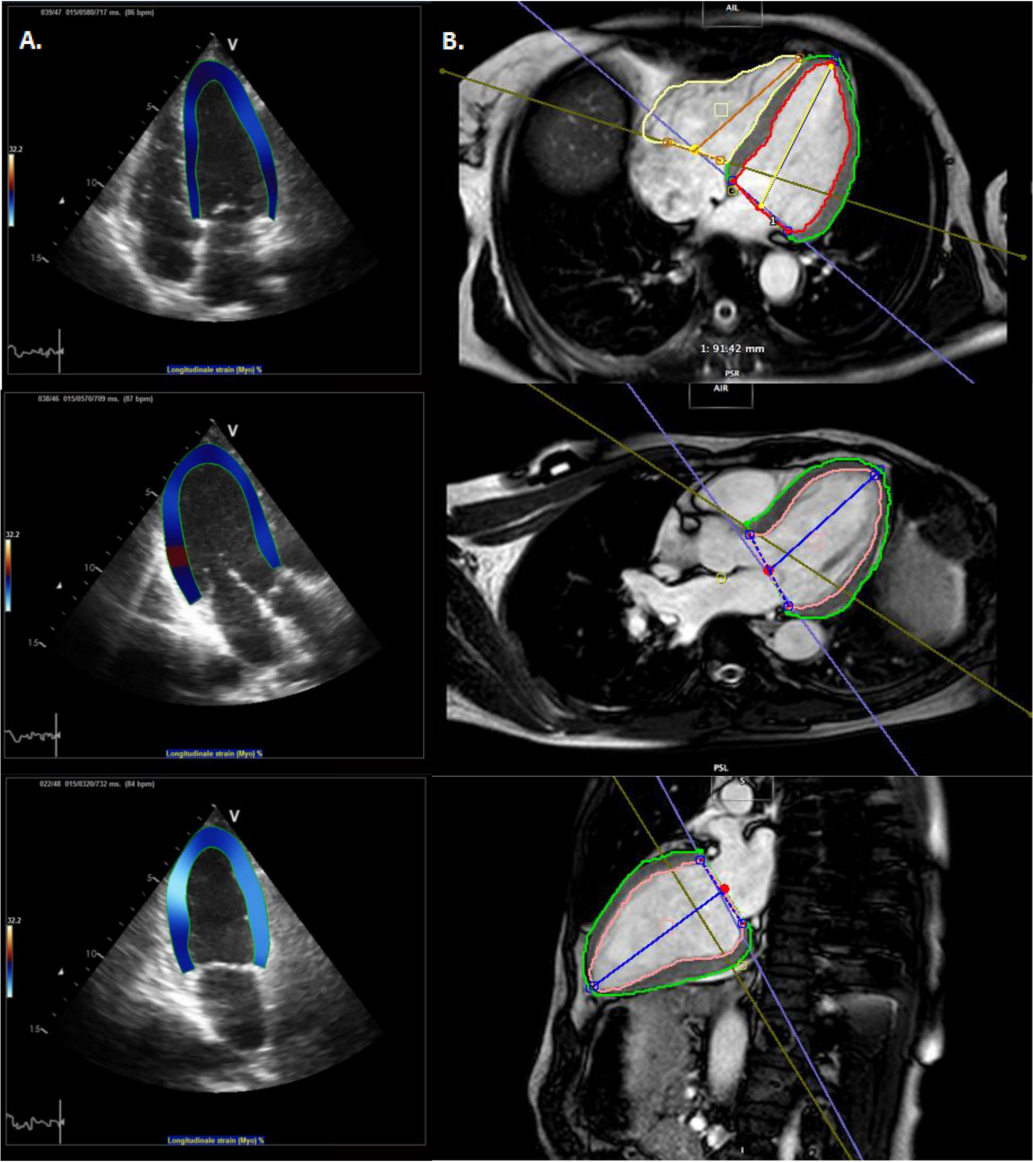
2D-STE and CMR images used for calculation of myocardial strain. Abbreviations: *CMR* cardiac magnetic resonance imaging, *2D-STE* two-dimensional speckle tracking echocardiography. A. Speckle tracking analysis with 2D-STE of apical 4-chamber, 3-chamber and 2-chamber view. B. Speckle tracking analysis with CMR of transaxial 4-chamber, 3-chamber and sagittal 2-chamber view
